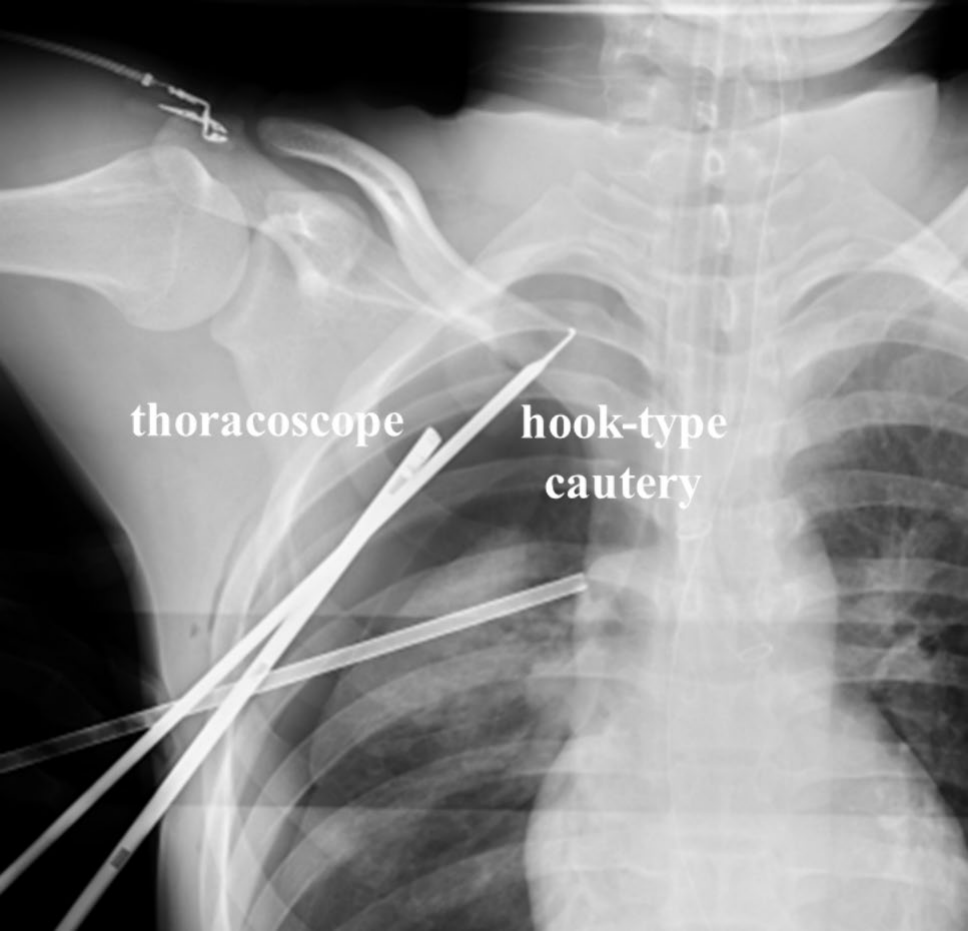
Intraoperative chest roentgenogram confirming the third rib
